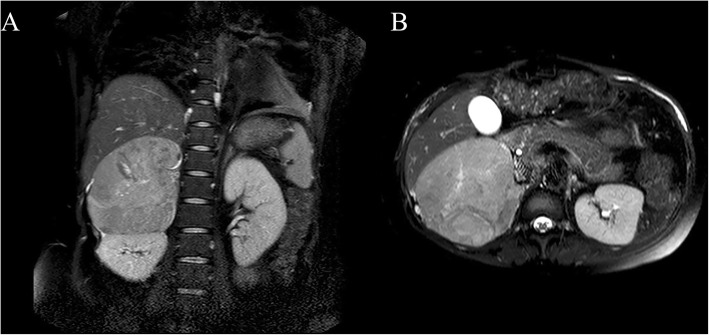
Coronal (a) and transverse (b) pelvic magnetic resonance imaging performed preoperatively demonstrates a soft tissue mass measuring 10.1 × 10.5 × 12.8 cm in the right retroperitoneal adrenal region, which oppressed the right kidney and liver
